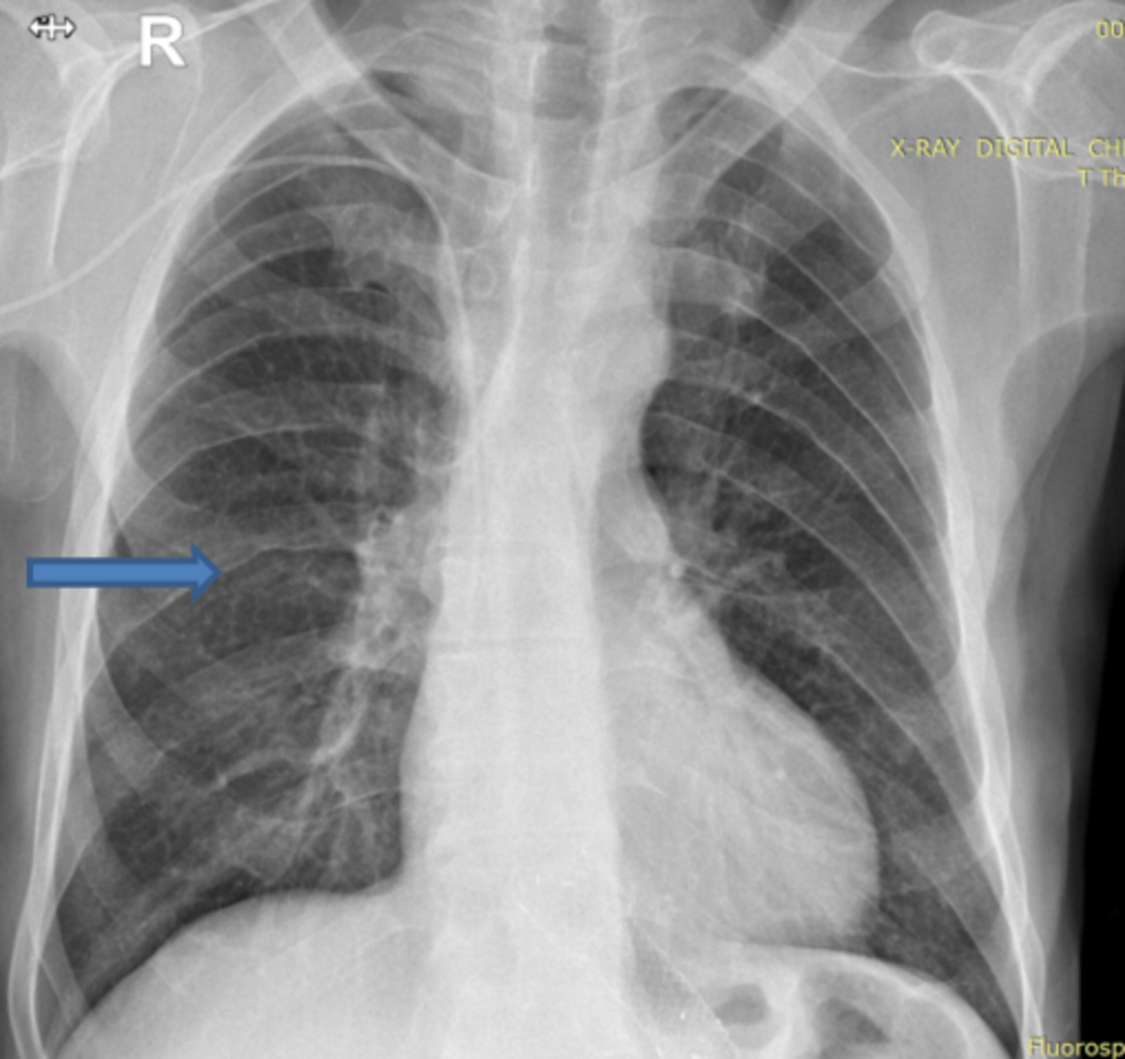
Chest X-ray revealing rib notching.
